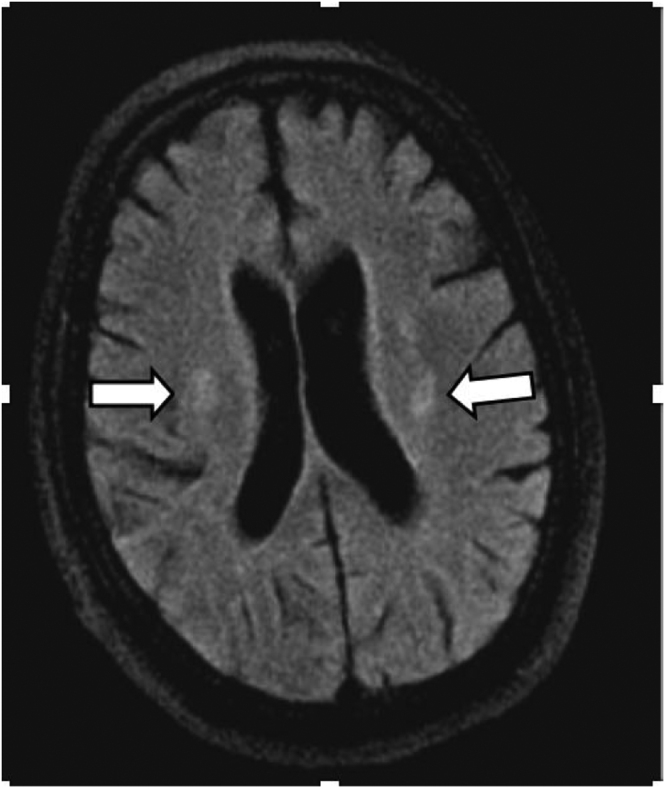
Initial non-enhanced MRI brain. Axial FLAIR sequence demonstrates multifocal symmetrical periventricular white matter hyperintensities (White arrows).
